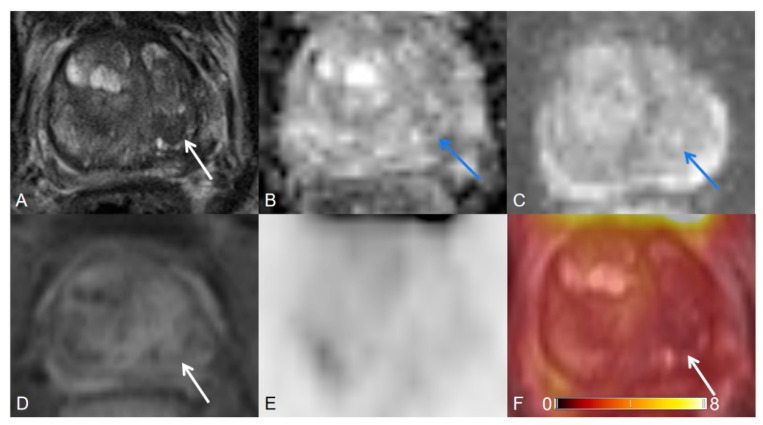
Transition zone with an atypical nodule. (A) Axial T2WI shows a T2 hypointense homogeneous circumscribed nodule. (B) ADC map image presents a focal lesion with a mildly hypointense signal below the background corresponding to the lesion seen in (A). (C) DWI (b = 1000 s/mm^2^) shows a focal lesion with a mildly hyperintense signal above the background corresponding to the lesion seen in (A,B). (D) Early dynamic contrast-enhanced image presents no early enhancement within the nodule. T2WI = 2, DWI = 3, DCE = negative, PI-RADS assessment category = 2. (E) PET image shows no [^68^Ga]Ga-PSMA-11 avid uptake. (F) [^68^Ga]Ga-PSMA-11 PET/MRI fusion.
